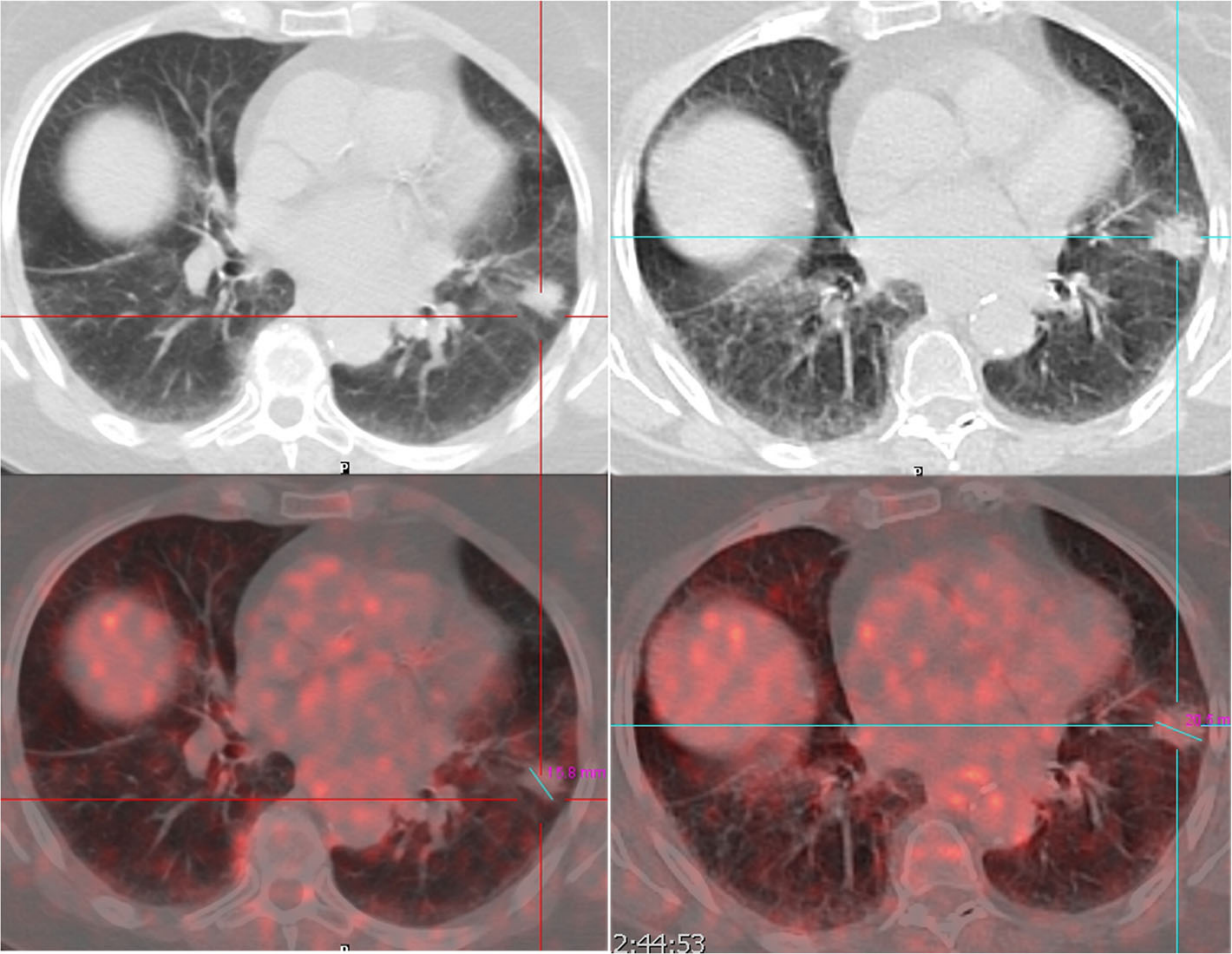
Left: baseline PET scanner, 15.6 mm lingular consolidation, no abnormal FDG uptake; right: follow up PET scanner, 15 months later, 20.5 mm lingular consolidation, no abnormal FDG uptake
